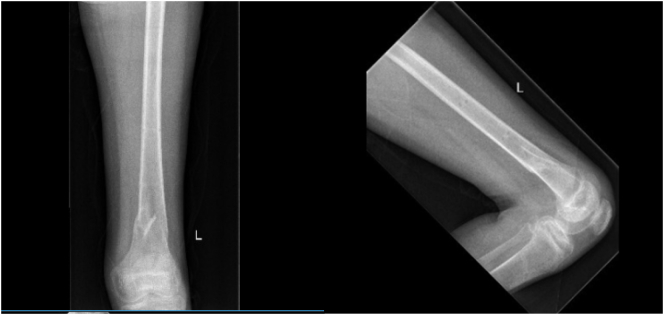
post-operative radiograph of the left distal femur after removal of external fixator (8 weeks).
